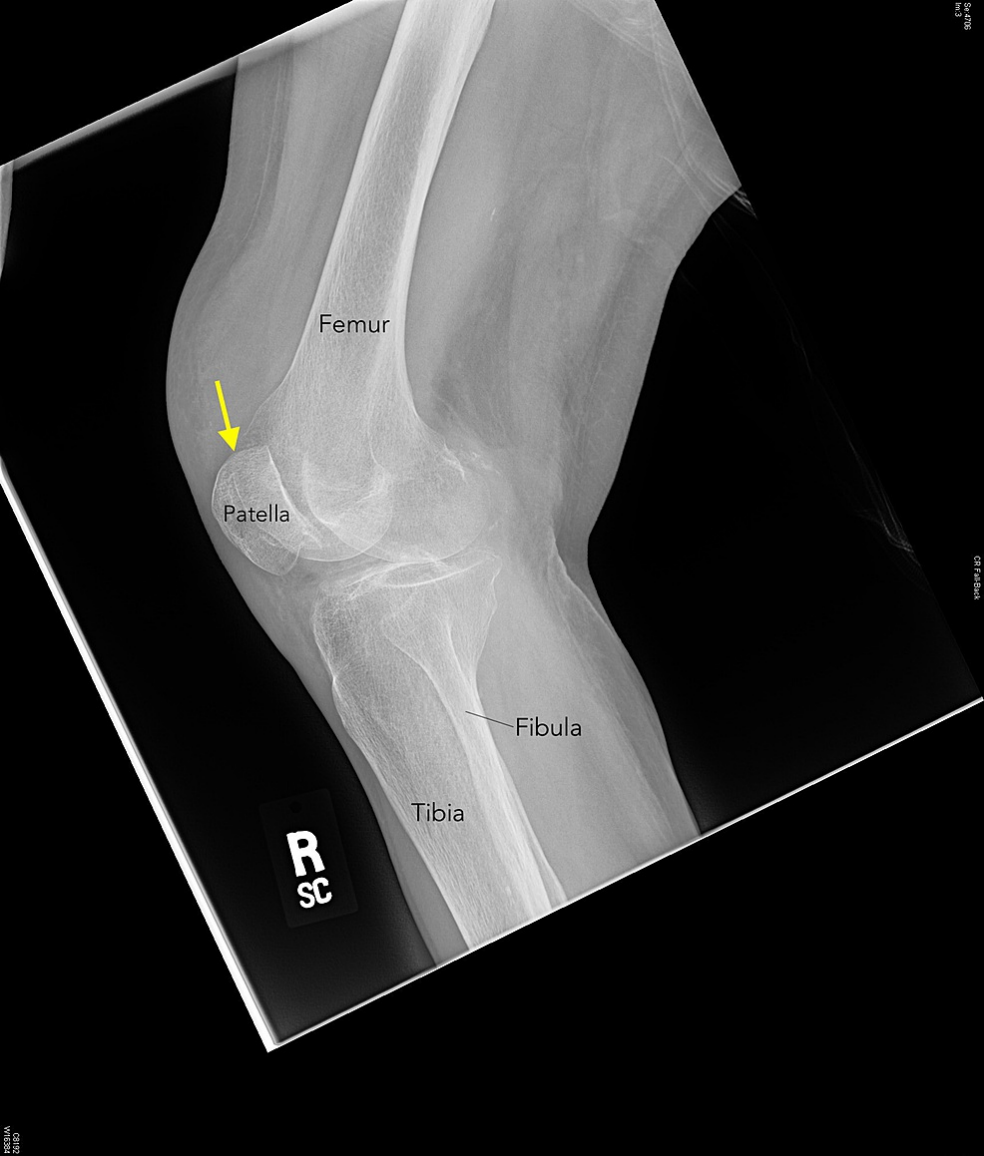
Knee radiograph demonstrating small joint effusion in the suprapatellar recess (arrow)
